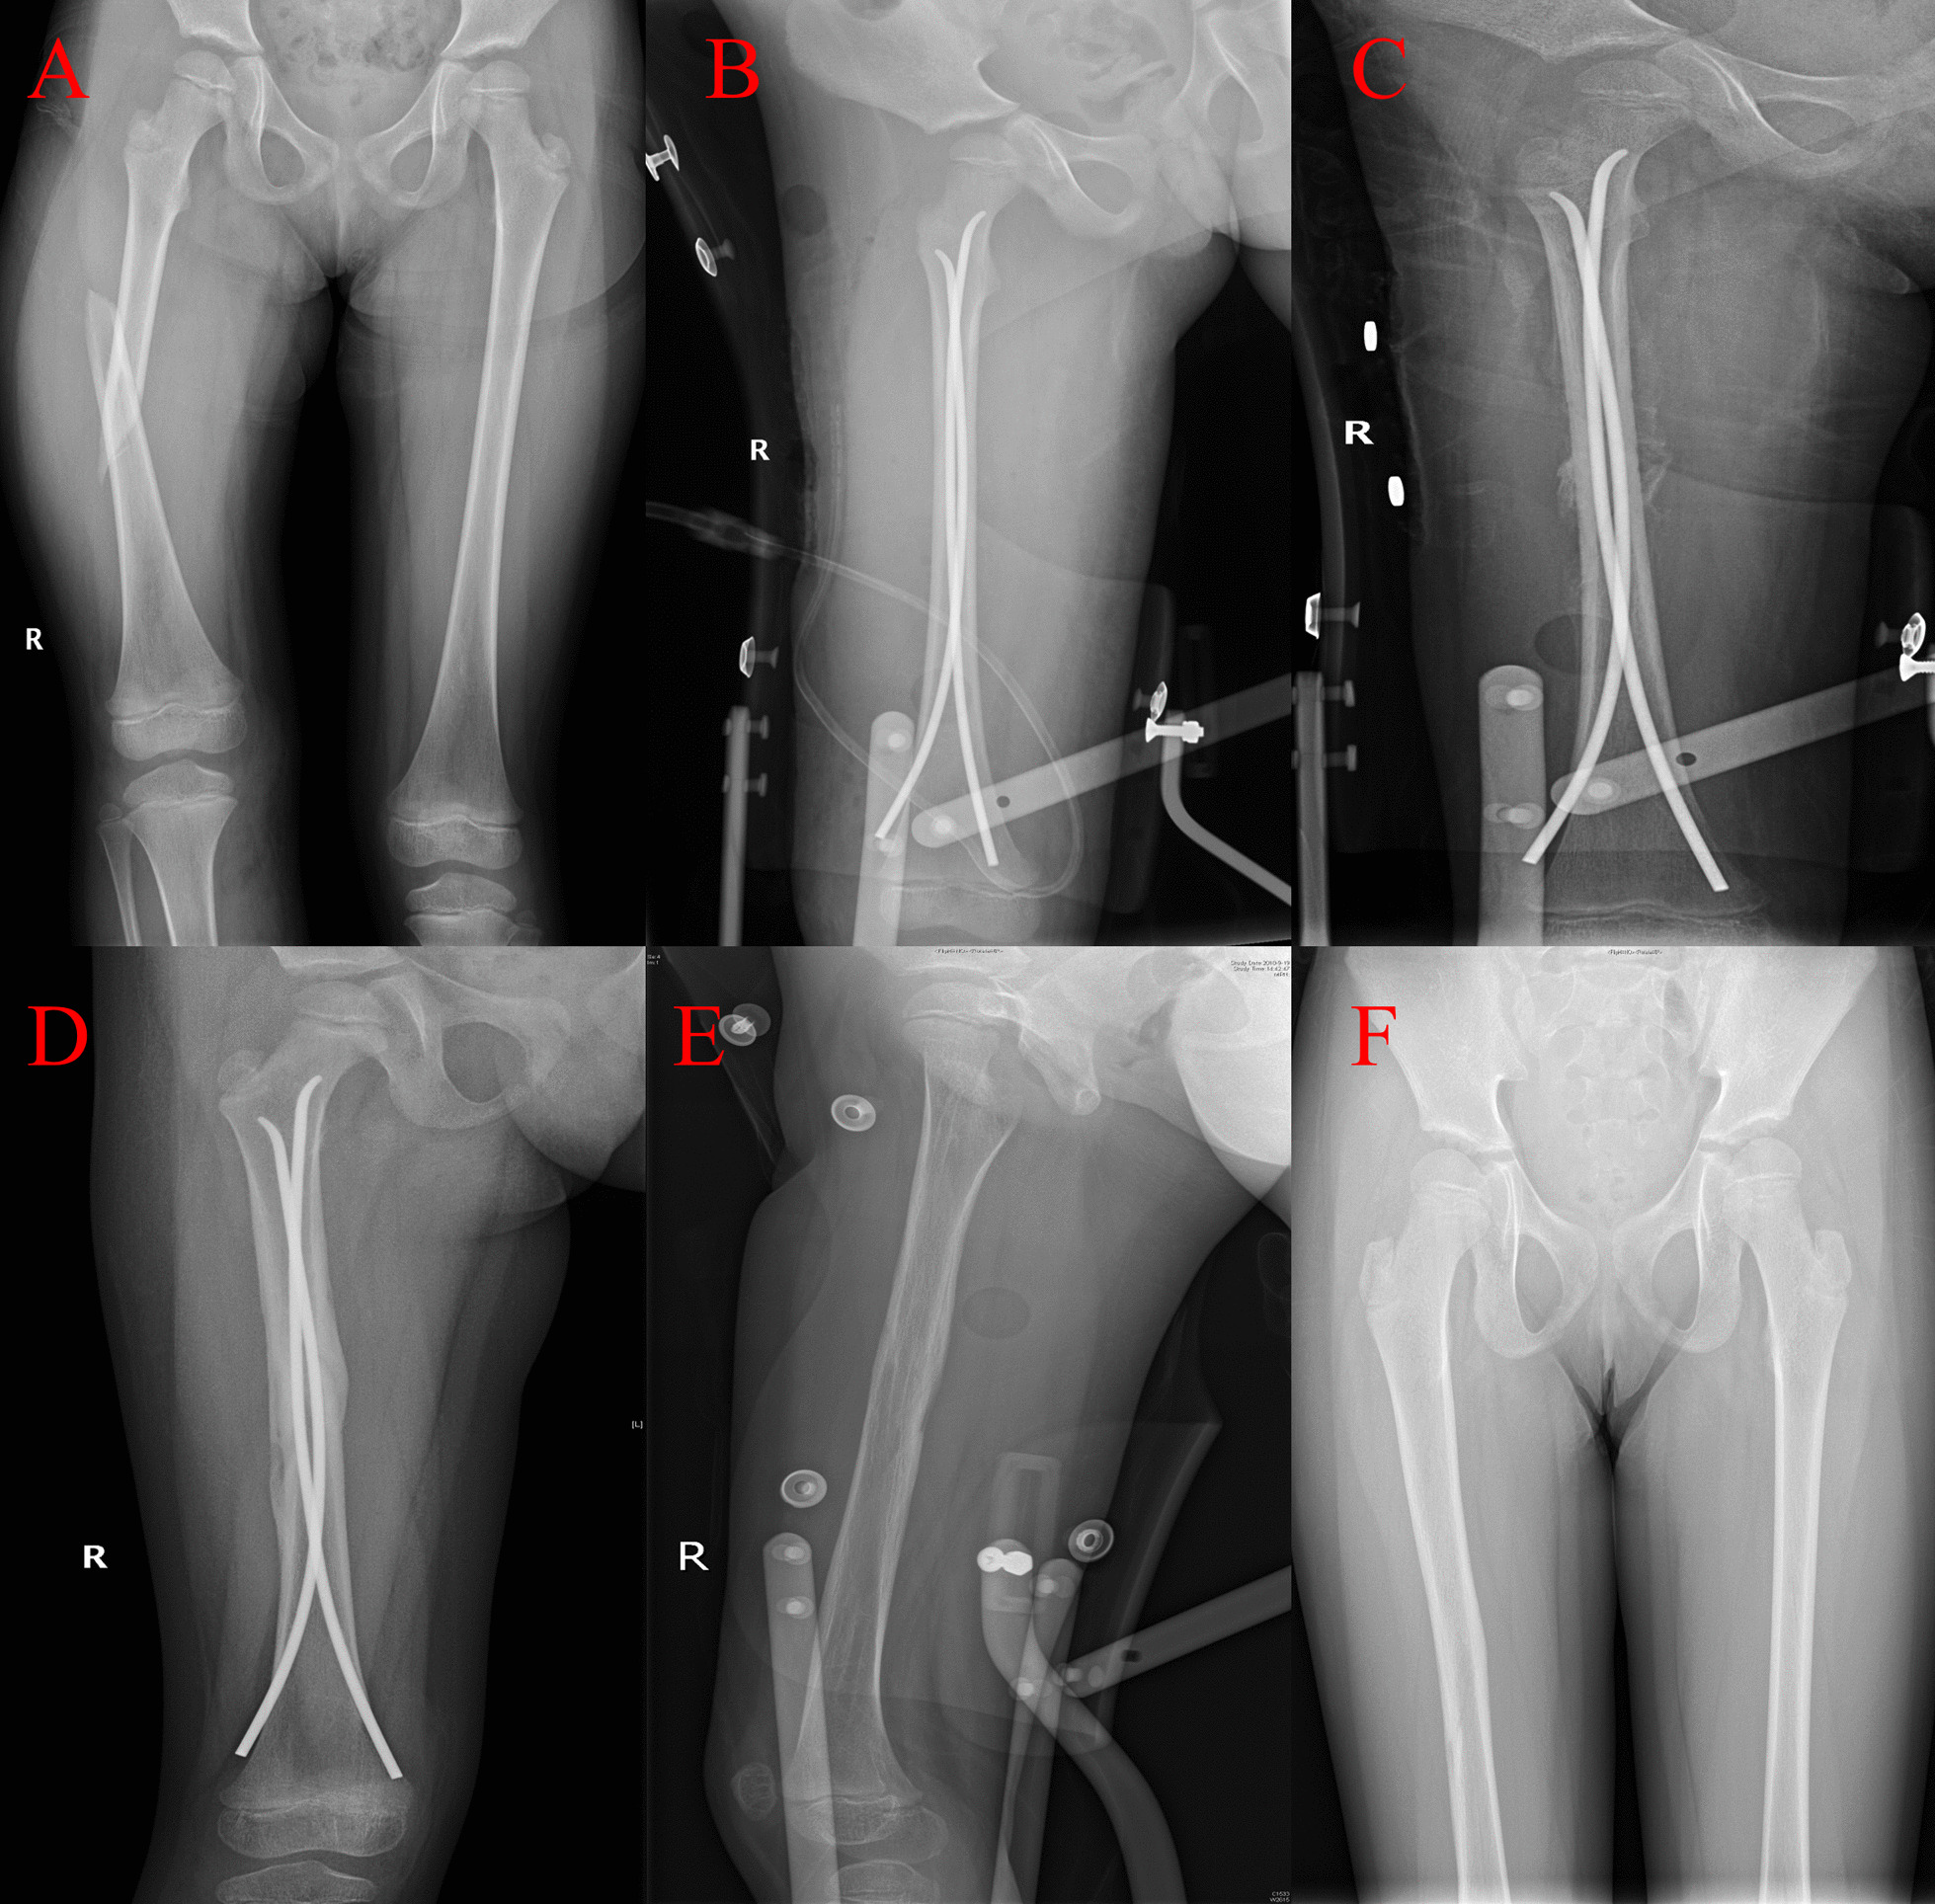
A 5-year-old male injured in a car accident and treated using FIN. A AP radiographs of the right femoral fracture caused by the accident. B Postoperative AP radiograph showing FIN fixation. C AP radiograph at 1 month postoperatively. D AP radiograph at 3 months postoperatively. E AP radiograph at 7 months postoperatively. F AP radiograph at 18 months postoperatively. *AP* Anteroposterior; *FIN* Flexible intramedullary nails
